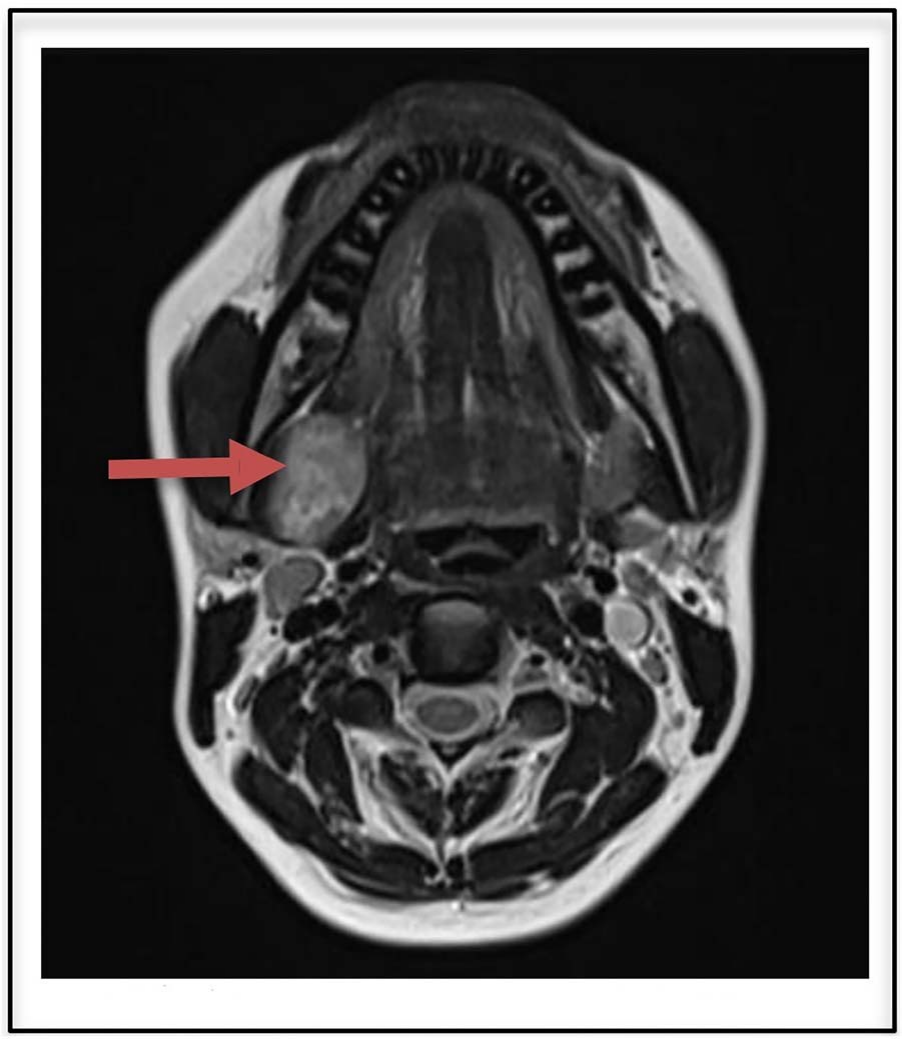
T2 heterogenously hyperintense oval shaped well circumscribed lesion measuring 2.9 × 2.7 cm on MRI (arrow).
